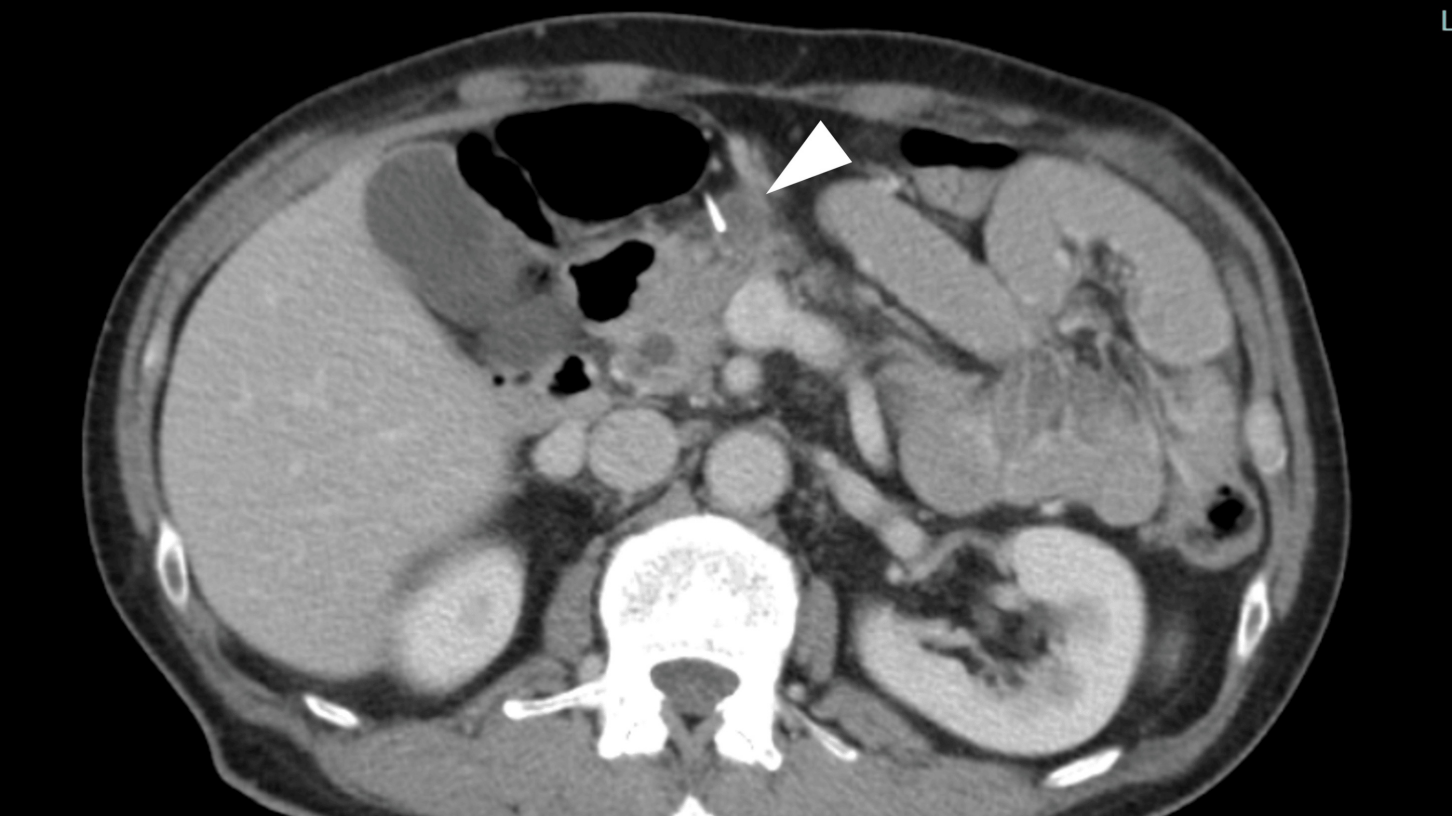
Axial abdominal contrast-enhanced computed tomography after four courses of pembrolizumab monotherapy Lymph nodes (arrowhead) shrank after four courses of pembrolizumab monotherapy.
